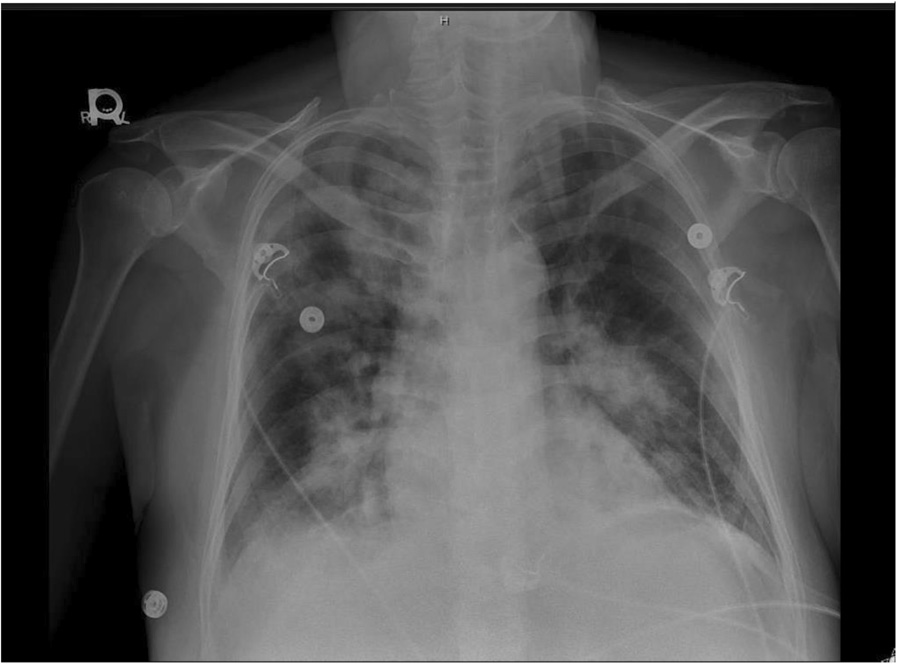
Chest X-ray, Portable film notable for bilateral, patchy hilar infiltrates consistent with pulmonary edema in the setting of depressed ejection fraction
